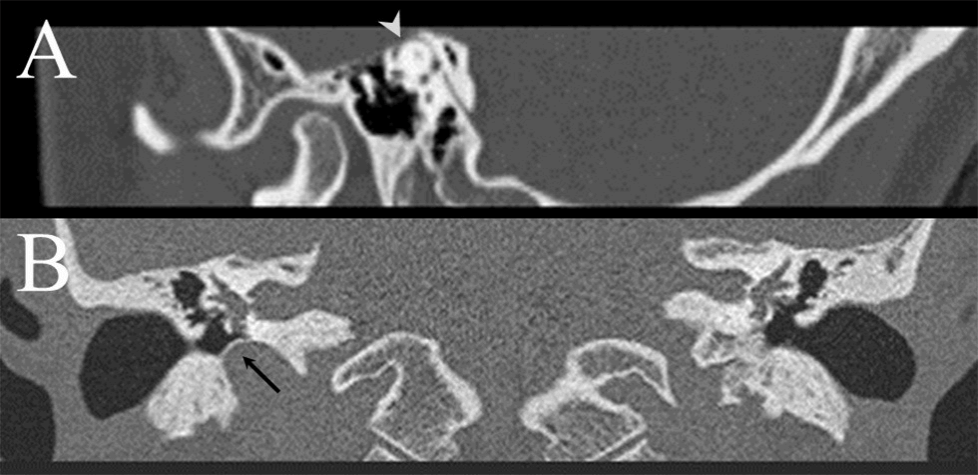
(A) Temporal bone CT demonstrates dehiscence of left superior semicircular canal in patient 4 (arrow head). (B) Temporal bone CT of patient 5 discloses high position of right jugular bulb with a proximity to the middle ear cavity and vestibular organ, with a thin sigmoid plate covering the roof of the jugular bulb (arrow).
